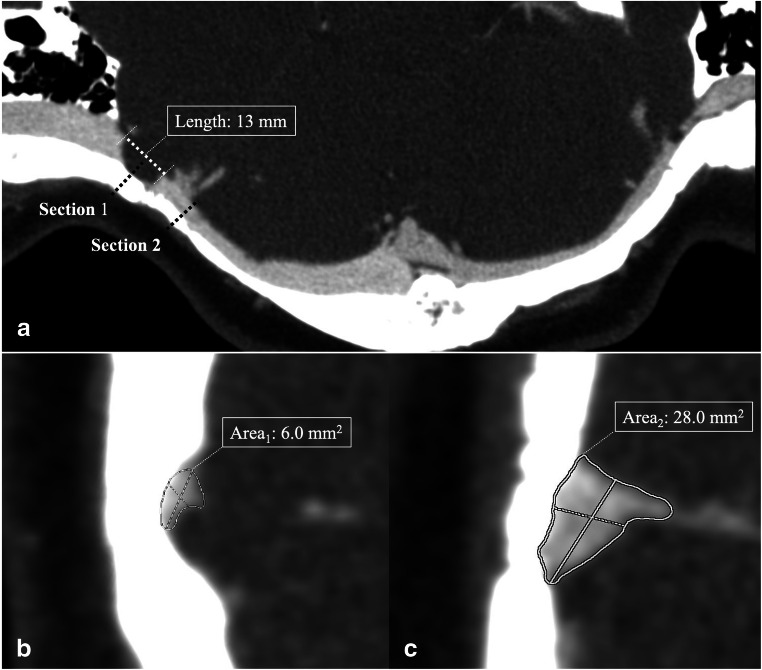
The degree and length of transverse sinus stenosis. The entire course of transverse sinus is shown in a curved reformatting CT image (a). The percentage of transverse sinus stenosis is calculated by dividing the cross-sectional area of stenosis (b) by the normative area of adjacent transverse (c). The range is also measured based on CT curved planer reformation
